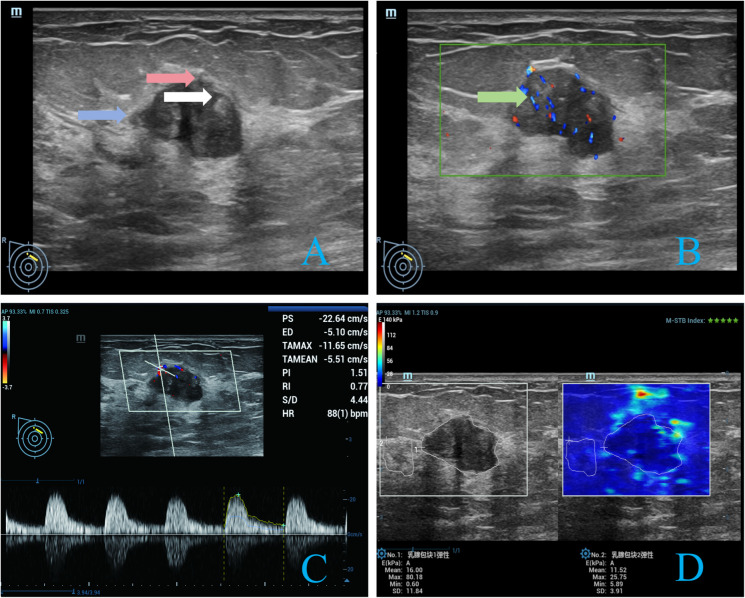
Multimodal ultrasound findings of the breast lesion (A–D). (A) Grayscale ultrasound shows an irregular hypoechoic lesion with indistinct margins, focal microlobulations, spiculated margins, and peripheral punctate echogenic foci. (B) Color Doppler imaging shows feeding vessels entering the lesion and internal vascular signals. (C) Pulsed-wave Doppler demonstrates an arterial waveform within the lesion. (D) SWE demonstrates heterogeneous stiffness distribution within the lesion, with increased stiffness compared with the adjacent normal glandular tissue. Blue arrows indicate microlobulations, pink arrows indicate spiculated margins, white arrows indicate punctate echogenic foci, and green arrows indicate vascular signals.
